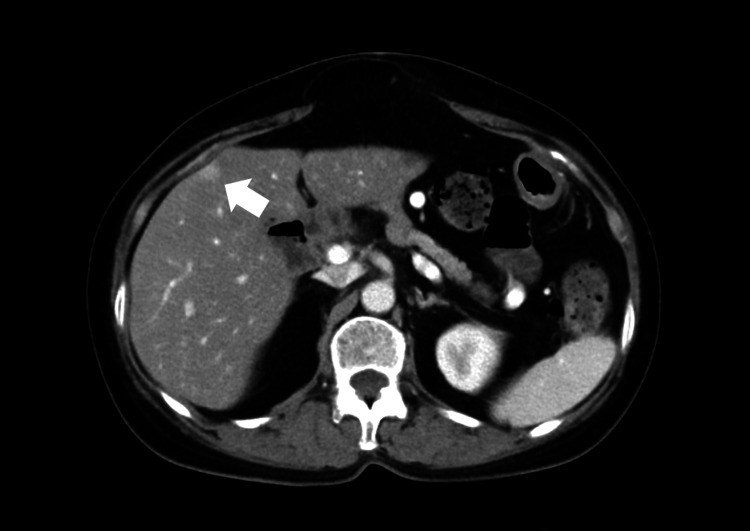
Computed tomography scan of Case 3 revealed a small early enhancement mass (arrow) on the surface layer in segment 5 of the liver.
